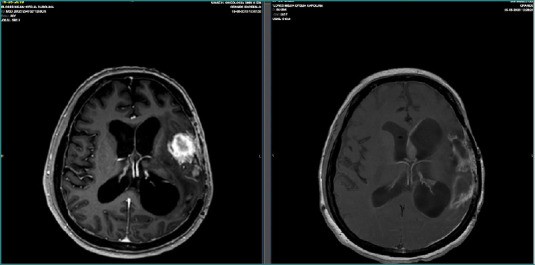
Axial preoperative and postoperative MRI scans obtained 8 months after multimodal treatment.
